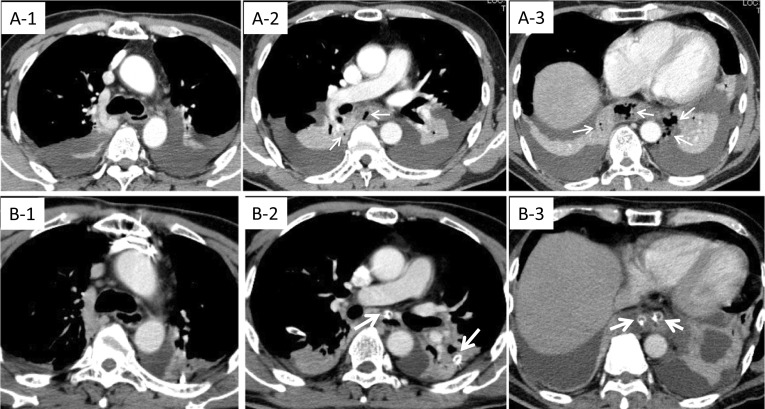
CT findings before (A-1,2,3) and after (B-1,2,3) an emergency operation. A1 -3. CT before emergency surgery. A-1 Pneumomediastinum is not seen in the branchial bifurcation. A-2,3 Pneumomediastinum is observed (arrow). B1-3 CT after 4 days from emergency surgery. B-1 Pneumomediastinum did not spread above the branchial bifurcation. B-2,3 The drain placed from the abdomen is effective in reducing pneumomediastinum and inflammation
